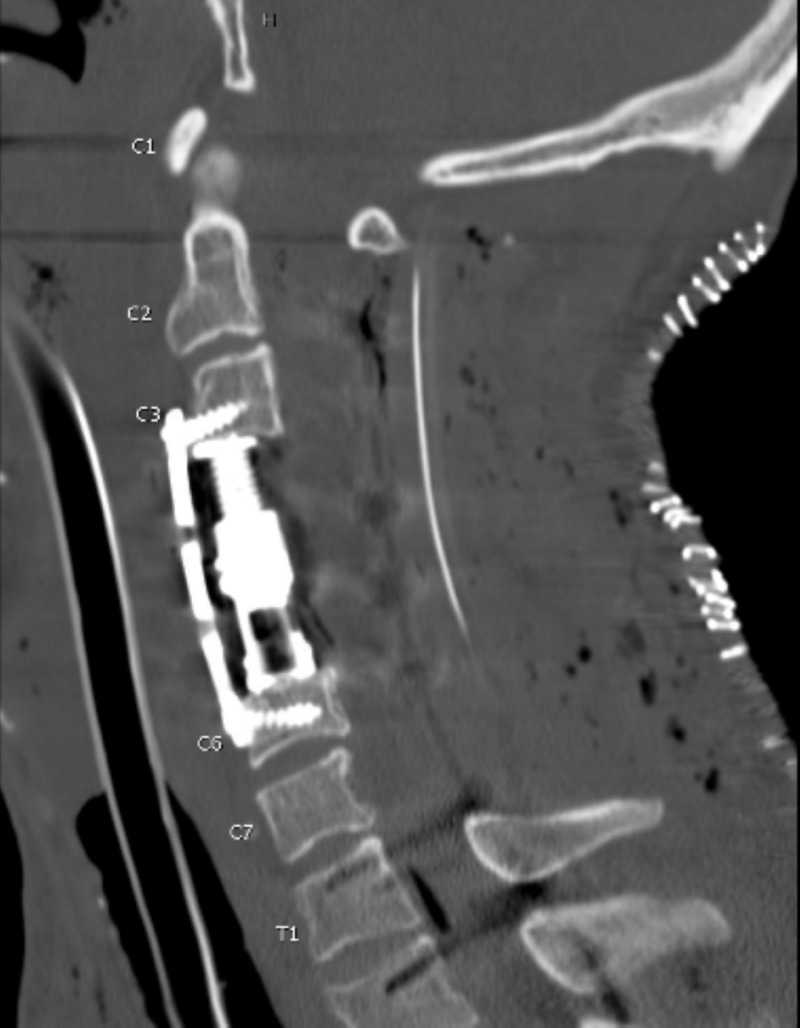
Post-operative sagittal CT non-contrast of the cervical spine Intubation tube is seen within the trachea. Postoperative changes including posterior drainage catheter and extensive subcutaneous emphysema are present. There is an anterior spinal fusion between C3 and C6 vertebrae with the corpectomy of C4-C5.
